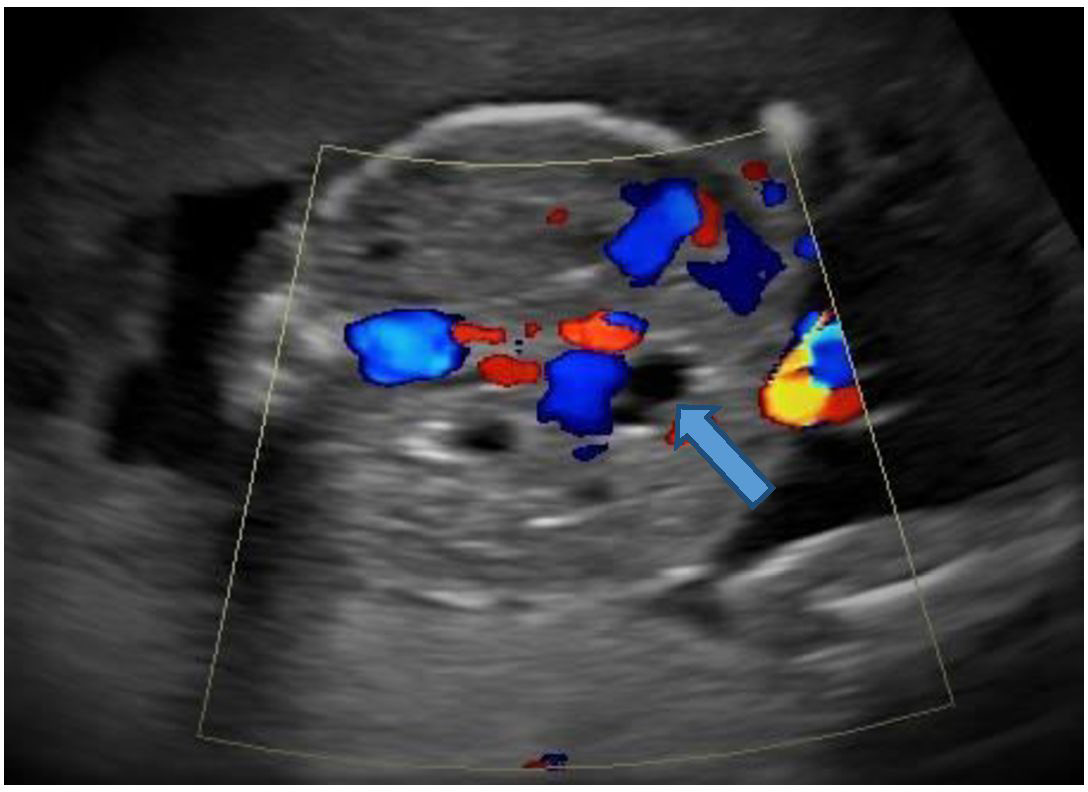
Color Doppler shows no color within the cystic structure (block arrow) in the liver suggesting a hepatic cyst.
